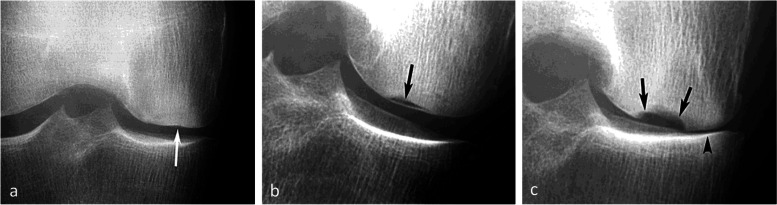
Evolution of an early subchondral insufficiency fracture with osteonecrosis. a Initial radiograph shows a slight hyperostosis near a discrete depression of the lower pole of the condyle (arrow). b Additional radiograph tangent to the posteroinferior pole of the condyle and performed with traction on the leg shows a typical subchondral bone dissection (arrow). c Three months later, this necrotic area shows a focal collapse (arrows), already complicated by a complete narrowing of the adjacent joint space (arrowhead)
